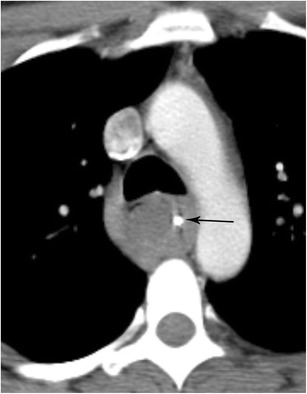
Hemangioma. CT angiogram of the chest in soft tissue window shows a round calcification within the wall of the oesophagus compatible with a phlebolith. This finding is most often seen in patients with varices, however this patient was found to have a hemangioma
